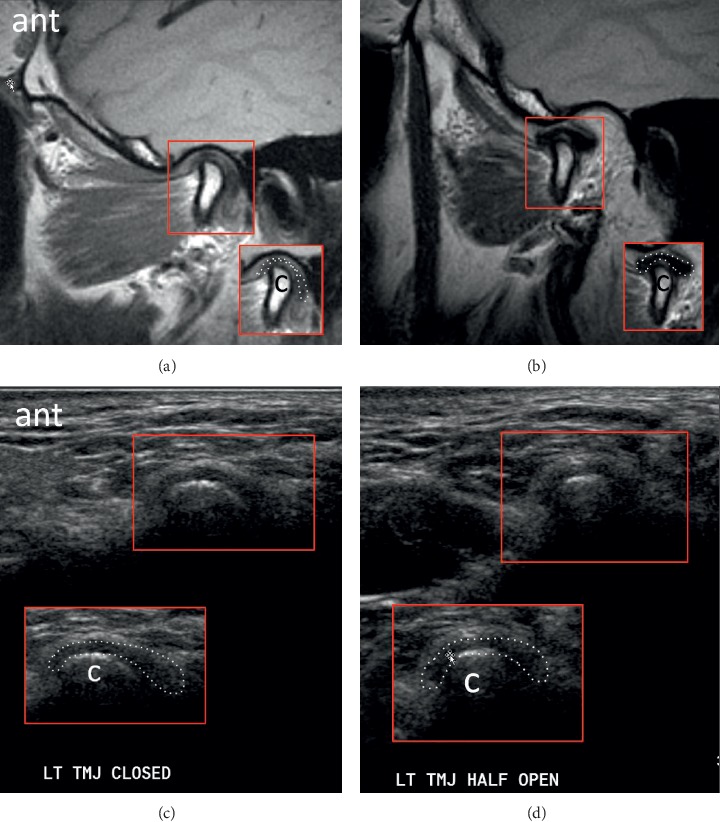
Images demonstrating posterior displacement of the disc in the (a) closed-mouth position with MRI, (b) recapture in the open-mouth position with MRI, (c) closed-mouth position with US, and (d) recapture in the open-mouth position with US. A focal annotated view was provided to aid visualization with the articular disc outlined with a dotted contour; anterior = “ant”; condyle = “c”; left = “LT.”
